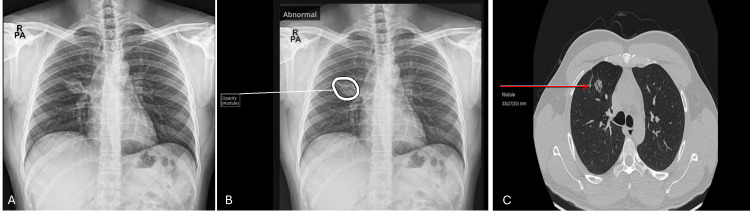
Chest X-ray and CT images of patient 4 A: Chest X-ray image without AI; B: Interpretation of chest X-ray with AI (suspicious nodules marked); C: Computed tomography (CT) image of the thorax (arrows show nodules)
